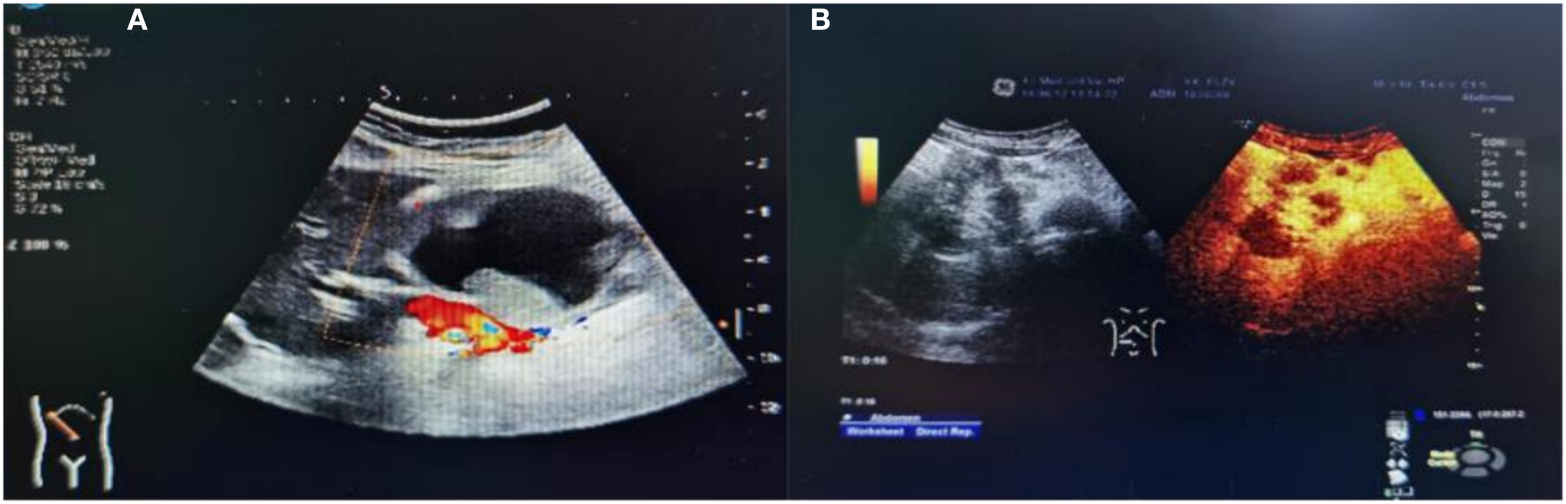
Ultrasound images of children with SPN. (A) Conventional ultrasound. (B) Contrast ultrasound.
